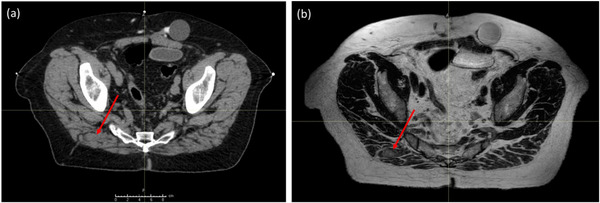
Transverse (a) CT and (b) MR images of a muscular metastasis. The tumor is visible on MRI but not on CT (red arrows).
